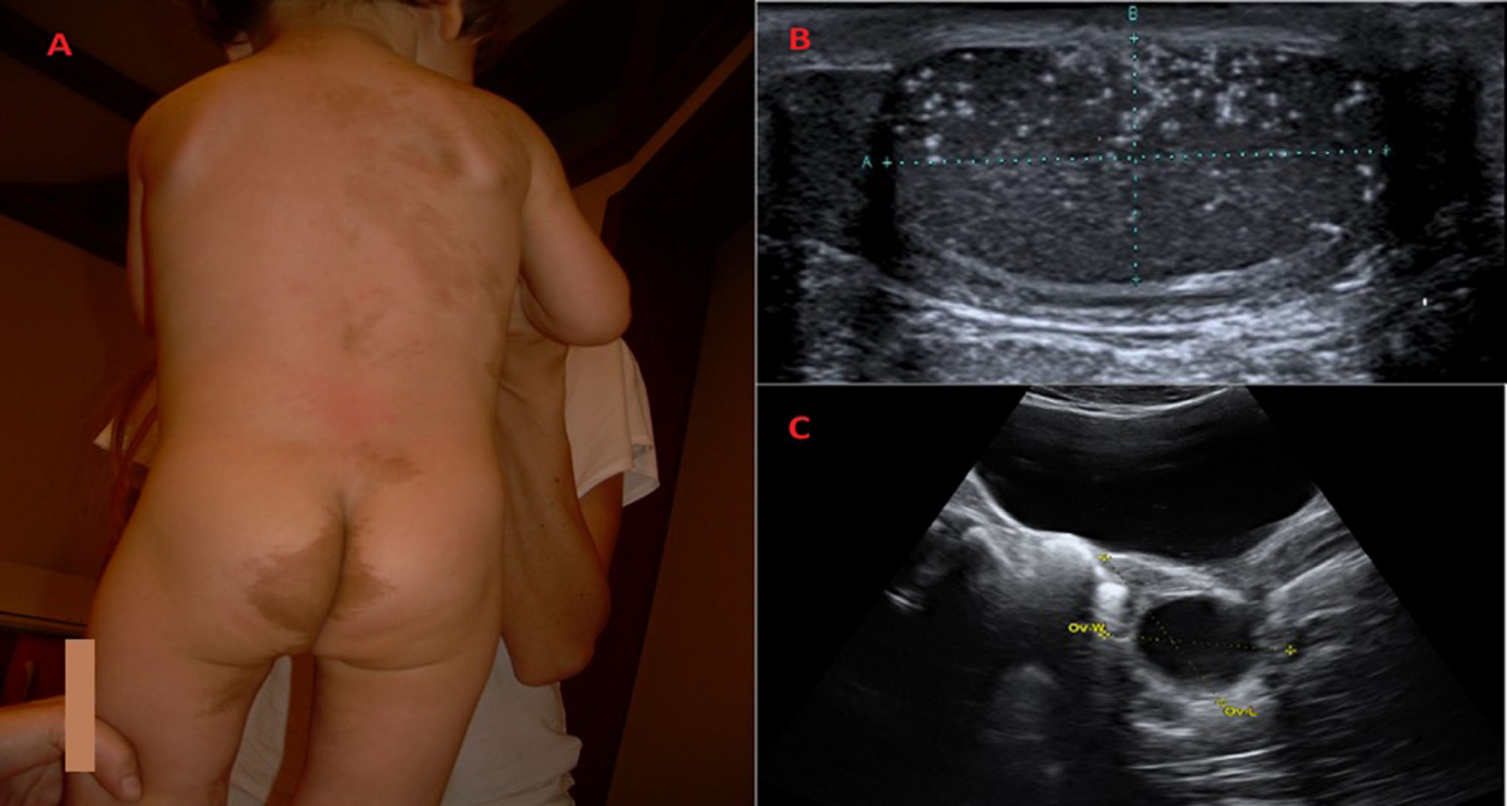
Clinical and radiological features of children diagnosed with McCune Albright syndrome. (A) Classic *Café-au-lait* skin pigmented lesion in a 25-months-old boy with McCune-Albright Syndrome. The spots with smooth borders, respect the midline and follow the developmental lines of Blaschko. At follow up, fibrous dysplasia of the both tibias has been revealed. (B) When he was 3-years-old, a light testicular asymmetry has been noted and testicular microlithiasis has been revealed on ultrasound. (C) Unilateral ovarian cyst in a 7-years-old girl with vaginal bleeding diagnosed with McCune Albright syndrome.
